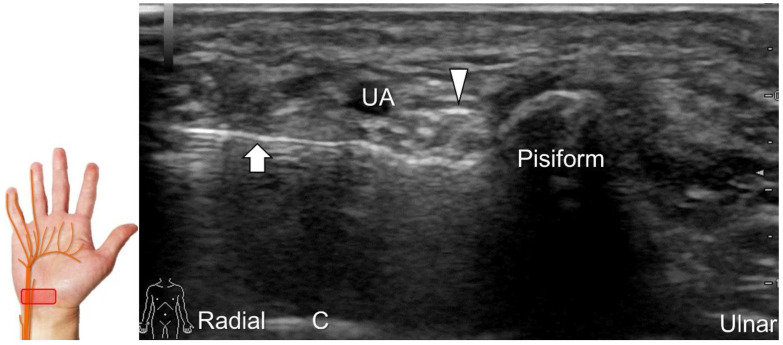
Ultrasound-guided injection of the ulnar nerve in short-axis view. Arrowhead: ulnar nerve; arrow: needle. UA: ulnar artery; C: capitate.
